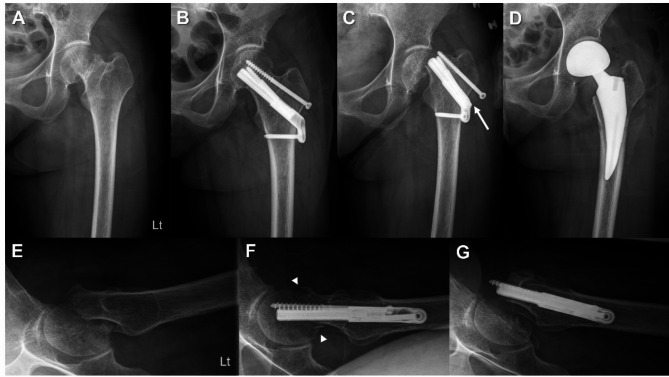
Case of a 48-year-old female femoral neck fracture (FNF) patient with underlying moderate-grade Intellectual disability. (A, E) The patient was diagnosed with Garden type 4 FNF, and the bone quality was poor due to general medical conditions. (B, F) In Immediate postoperative x-rays, the position of the bolt was not central, and posterior angulation of the femoral head was noted. We inserted an additional screw to maintain the reduction status and to compensate for the rotation instability. (C, G) Fixation failure occurred 1.5 months after the operation. The bolt slid laterally to a maximum degree (20 mm), and the additional screw was also pulled out. (D) We performed hemiarthroplasty after implant removal at 1.6 months after the initial surgery
